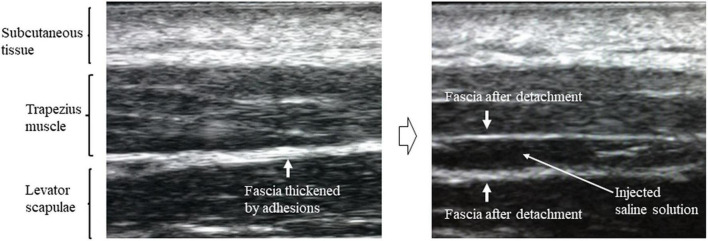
Ultrasound image showing thickening of the fascia between the levator scapulae and trapezius muscles before hydrodissection and removal of fascia after hydrodissection.
